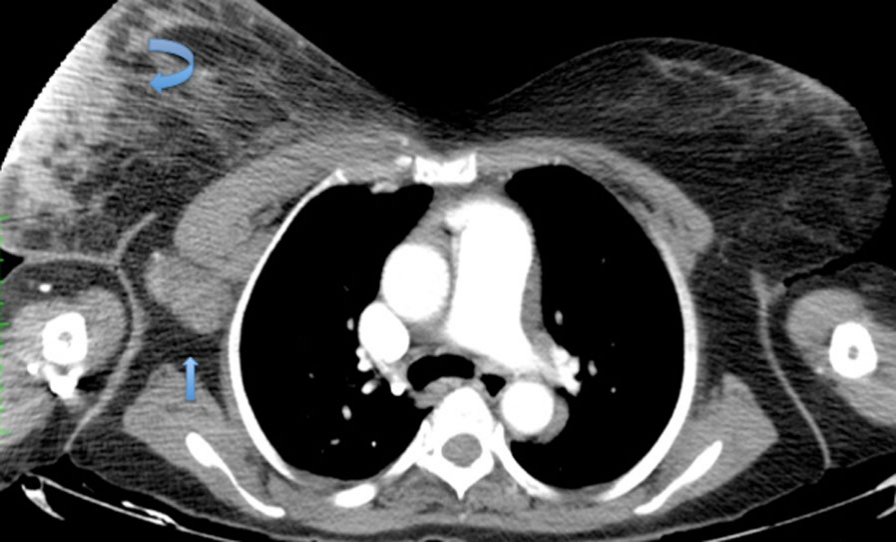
Axial contrast enhanced CT of the Chest. The right breast lesion was partially imaged (*curved arrow*) pre-operatively along with skin thickening and level I and II axillary adenopathy (*straight arrow*)
